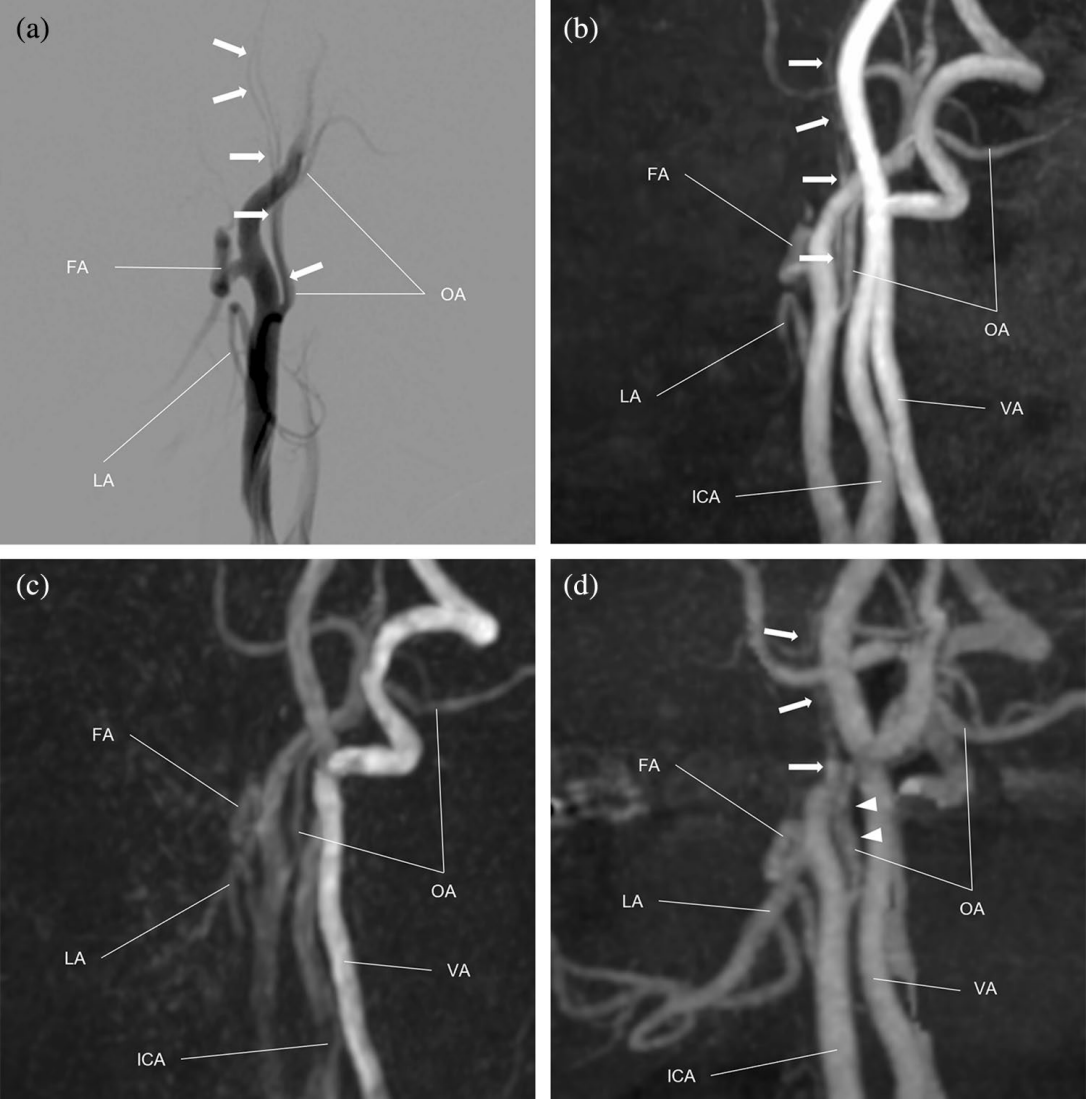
A 65-year-old male with left oropharyngeal cancer. (a) An early phase of the angiogram at the left external carotid artery. The ascending pharyngeal artery (APhaA) arises at the origin of the occipital artery and almost directly runs superiorly (arrows) (b) TOF-MRA demonstrates the APhaA entirely (arrows) and is rated as a score 5. (c) ASL-MRA does not demonstrate the APhaA at all (score 1). (d) CTA also demonstrates the APhaA as well as TOF-MRA (score 5). There are, however, it is hard to differentiate the proximal of APhaA from OA (arrow heads), so that the visualization quality for APhaA is slightly less than that of TOF-MRA.
